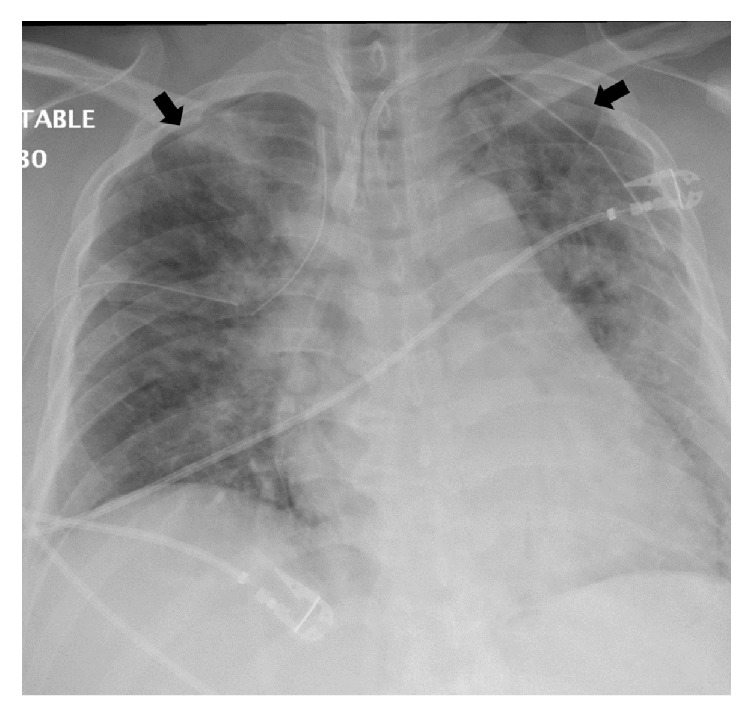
X-ray on postoperative day 1 after extubation. The patient was extubated on postoperative day 1 as he met extubation criteria. Shown is the X-ay after extubation, indicating that the bilateral pneumothoraces had increased despite bilateral chest tubes.
